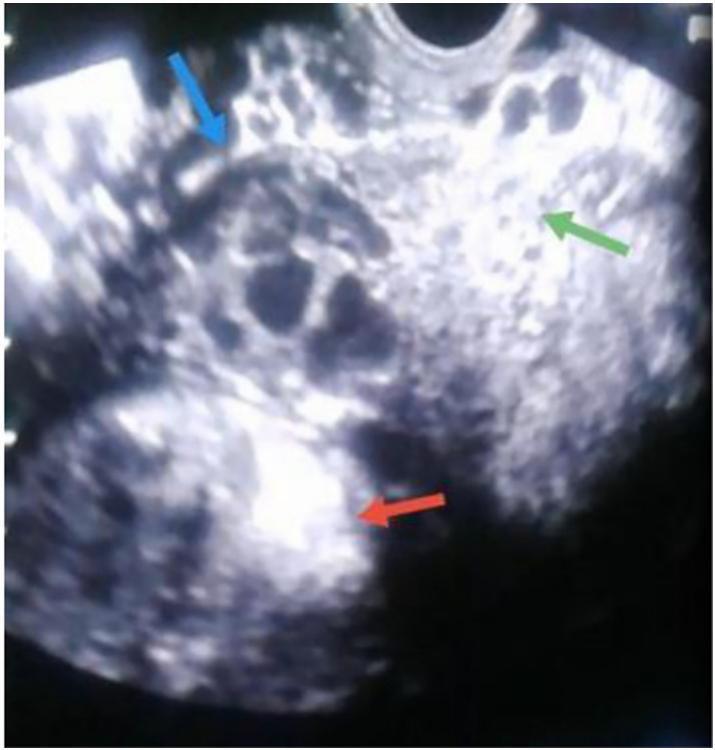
Transvaginal ultrasound (a retrospective diagnosis). Uterus (green arrow), rudimentury horn (blue arrow), placenta (red arrow). (For interpretation of the references to colour in this figure legend, the reader is referred to the web version of this article.)
